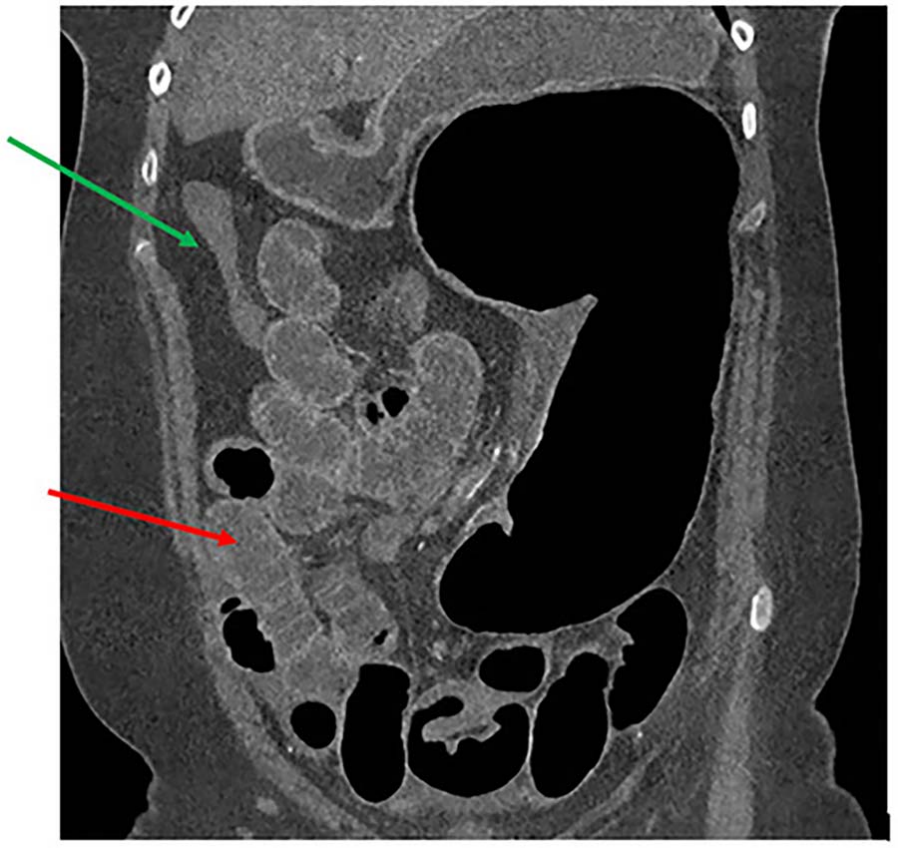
CT thorax, abdomen and pelvis coronal view demonstrating a distended, twisted caecum, caecum in the left upper quadrant, small bowel distension (red arrow) and a decompressed ascending colon (green arrow).
